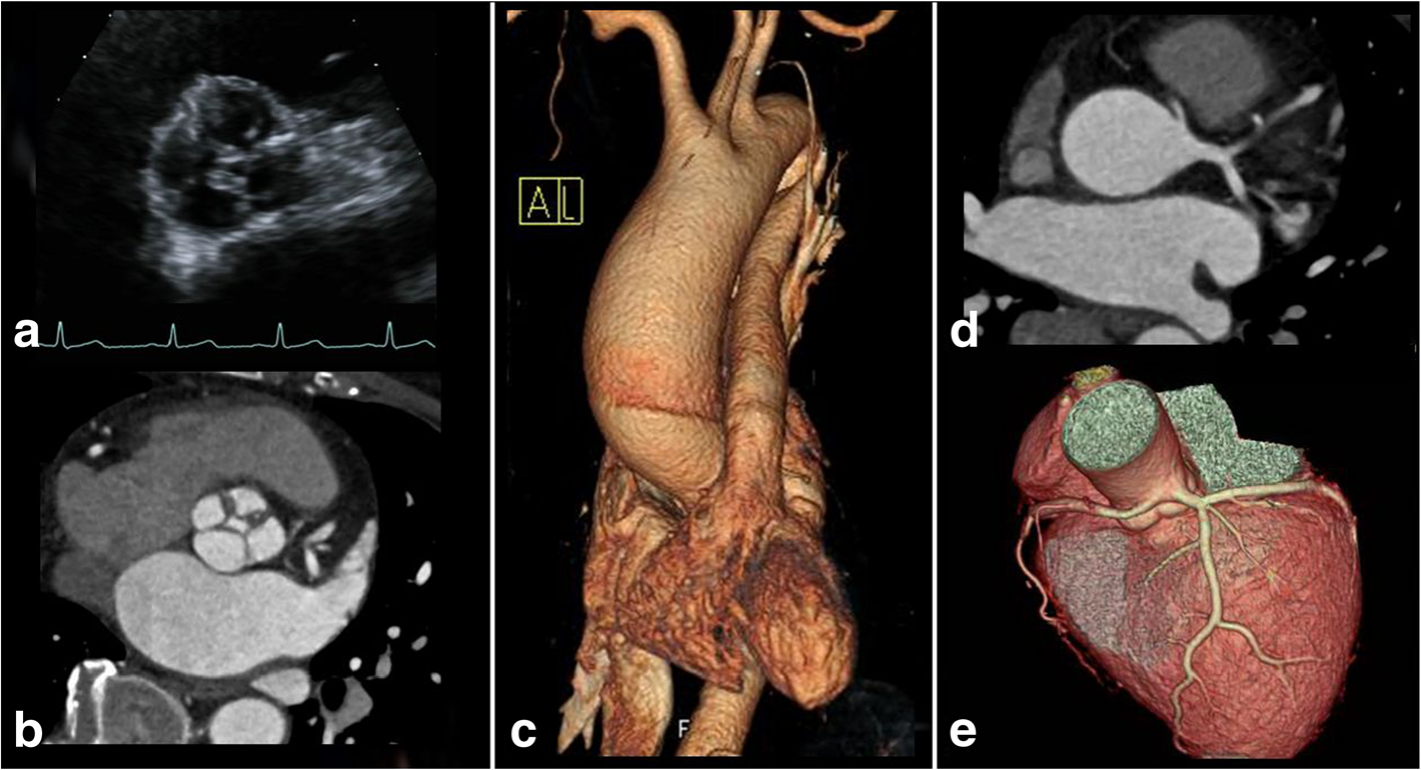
a. Transthoracic echocardiography showing a quadricuspid aortic valve with an ‘X-shaped’ commissural contour in the diastole. b. Computed tomographic angiography showing an aortic valve consisting of four symmetrically-sized cusps and a central coaptation defect resulting in central aortic regurgitation. c. Three-dimensional reconstruction imaging of computed tomography showing aneurysmal ascending aortic dilatation (maximum diameter = 45 mm) with preservation of the normal dimensions of the aortic root. d. and e. Volume-rendered multidetector computed tomography showing the spatial and anatomical relationships of the aortic cusps and the oval-shaped, single united coronary ostium
